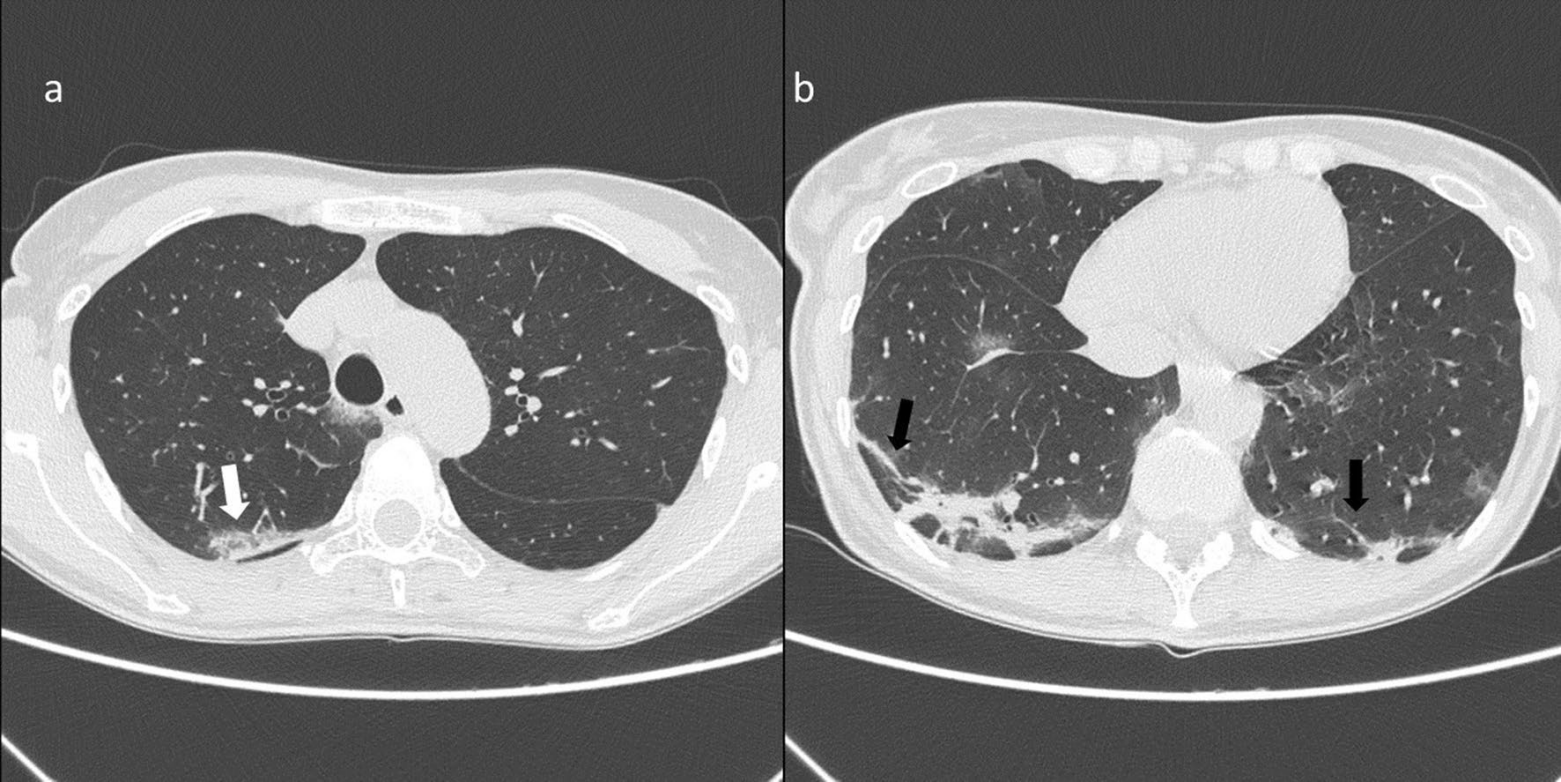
(a) CT scan shows focal consolidation in the subpleural area of the right upper lobe (white arrow); (b) CT scan shows bilateral areas of consolidation in the lower lobes with peripheral distribution and reticular pattern with the presence of fibrous stripes (black arrows).
